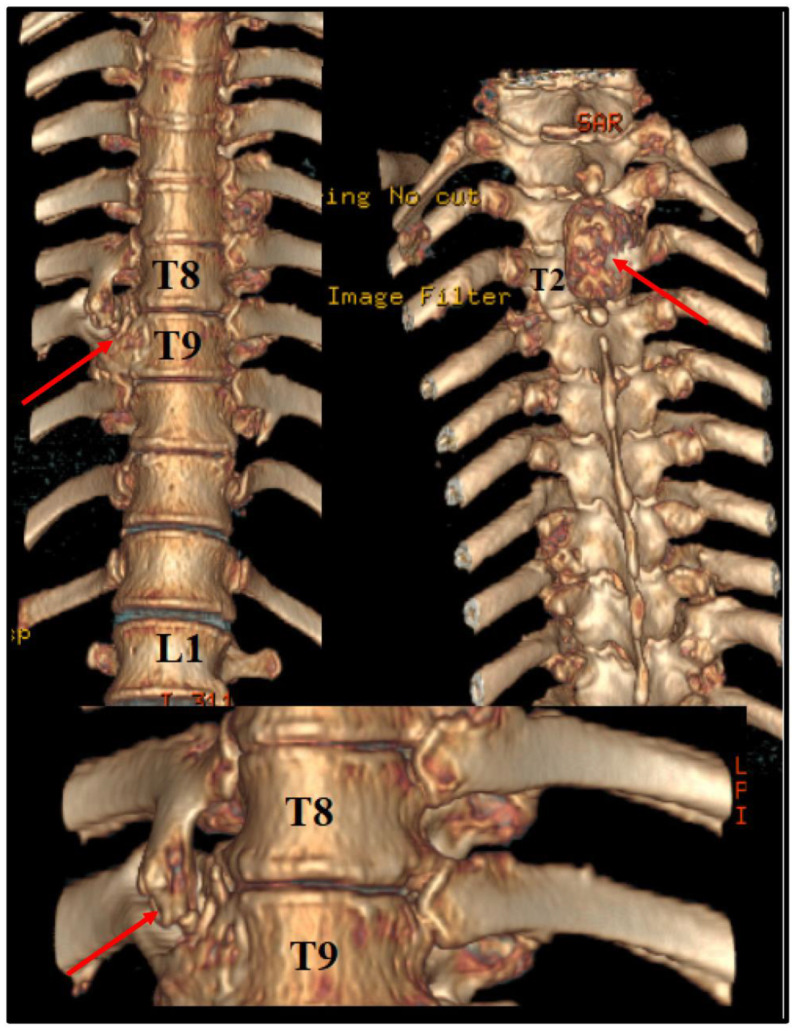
Preoperative 3D CT scan. Note the outer margins of the osteochondroma, along with another one, localized at T2 level, which was neurologically silent.
